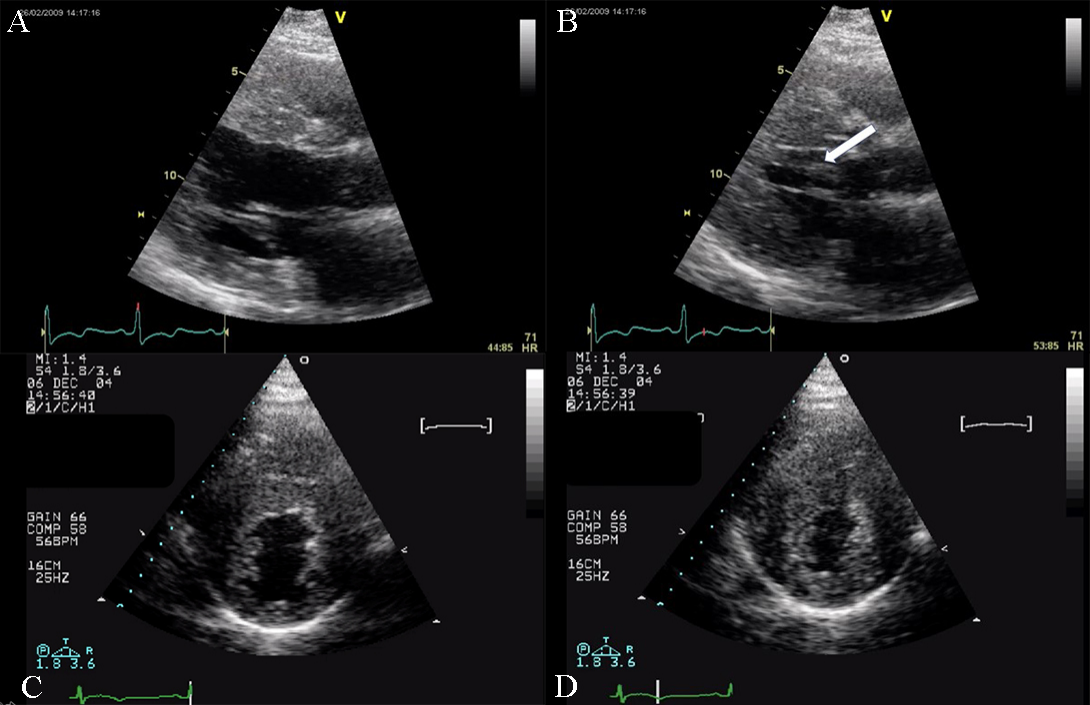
Echocardiographic aspect of HCM. (A) Parasternal long-axis view
